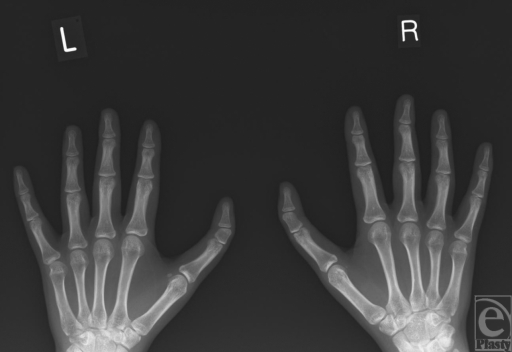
Preoperative radiograph of both hands showing the delta phalanx of the triphalangeal thumbs.
